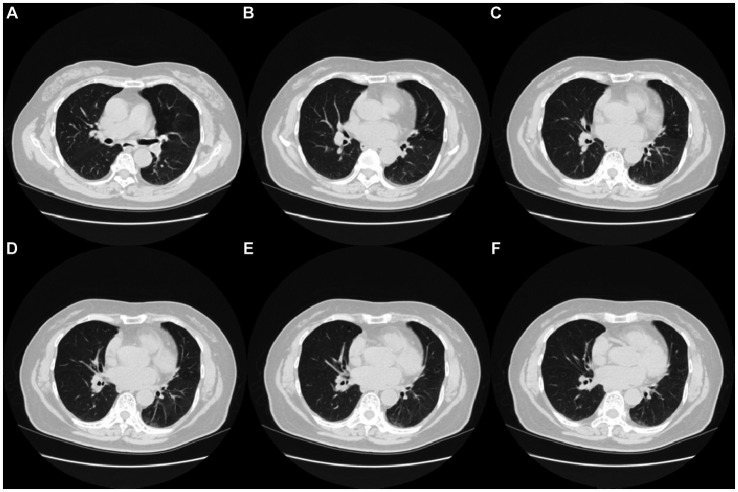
CT image results in lung infection in the patient (A–F) CT images of lung infection (Date 27-March-2023).
